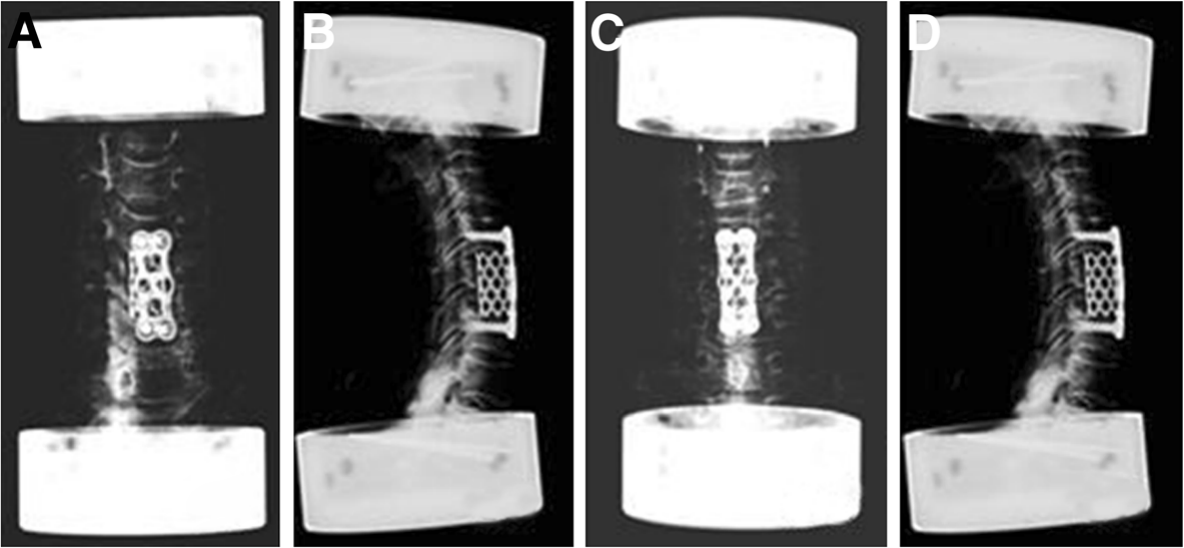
Postoperative anteroposterior and lateral radiographs of the anterior cervical corpectomy and the fusion of C5. (A, B) The novel self-locking plate system and (C, D) the DePuy plate system.
